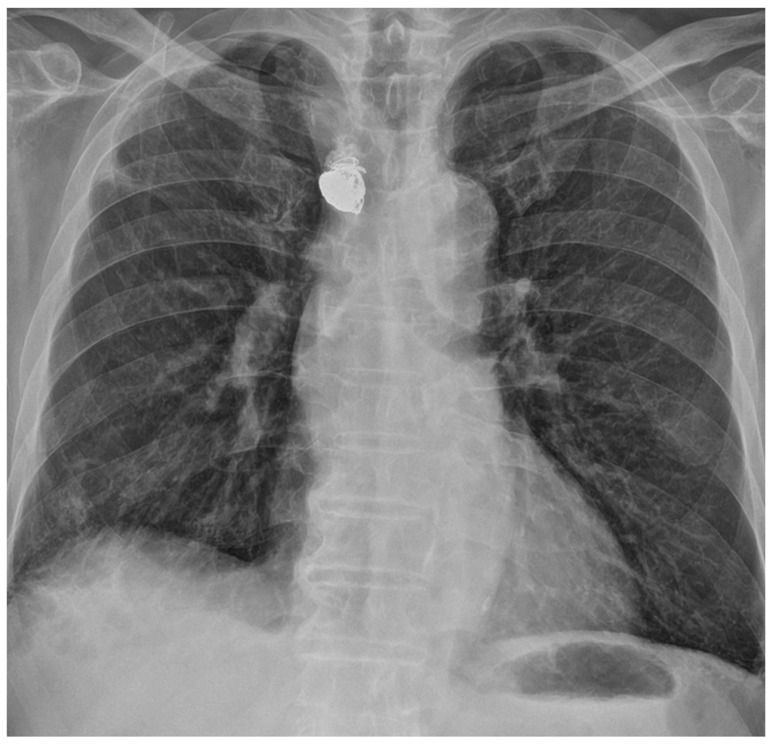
Chest radiographs acquired 10 months after the procedure show stable state of coils and N-butyl cyanoacrylate (NBCA) cast material.
